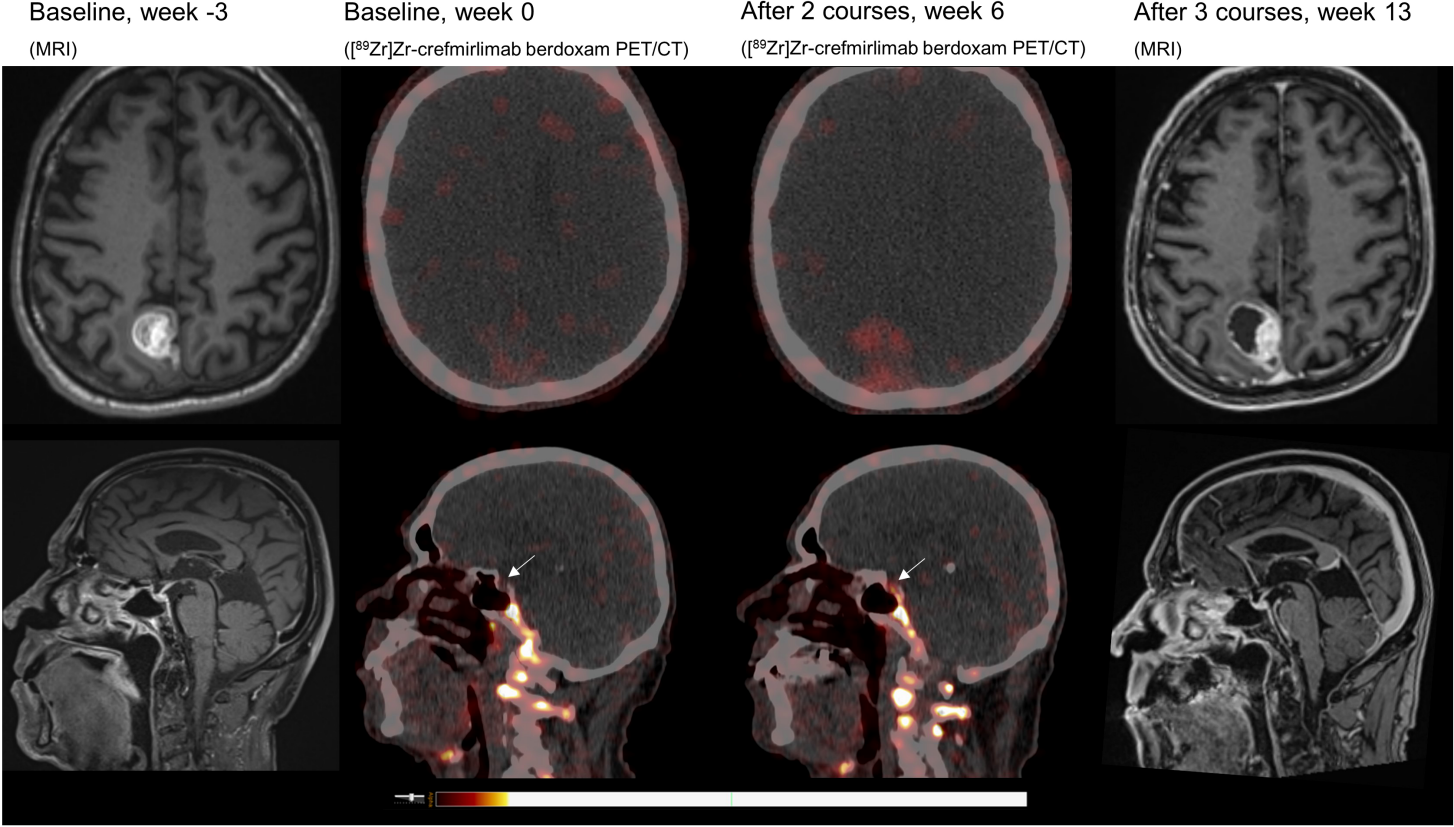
MRI and [^89^Zr]Zr-crefmirlimab berdoxam PET/CT scans of cerebral lesion and pituitary gland.
